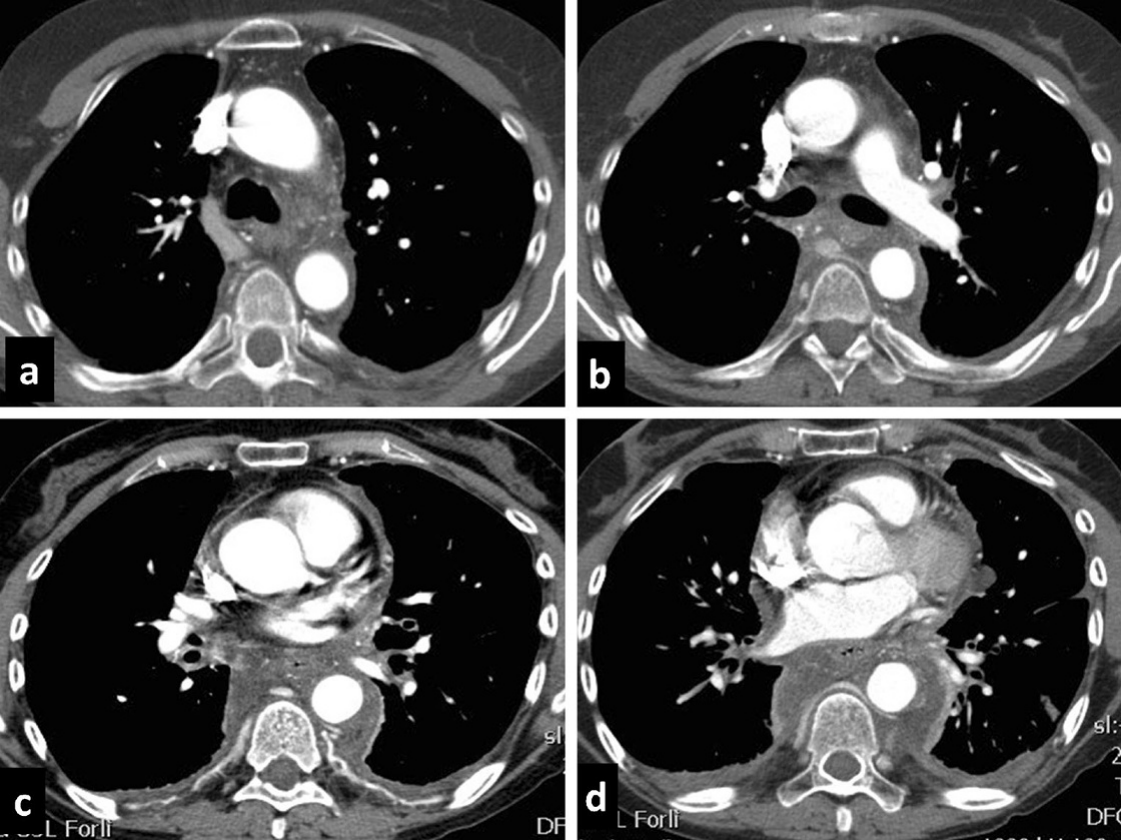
IgG4-related fibrosing mediastinitis. A 60-year-old female with a history of recurrent bilateral pleural effusion in the last 5 years. Clinical history of non-differentiated collagen vascular disease treated with steroids. CT showed marked hypertrophic tissue of the mediastinum and of the pleural space characterised as IgG4 sclerosing disease. A significant hypertrophy of the bronchial arteries (a–c) and intercostal vessels (c) can be observed. The azygos vein diameter is normal before anastomosis with the vena cava, whereas it shows a compression of the lumen in the medio-thoracic tract. Hypodense tissue is seen in the pleural space at the costophrenic angles
